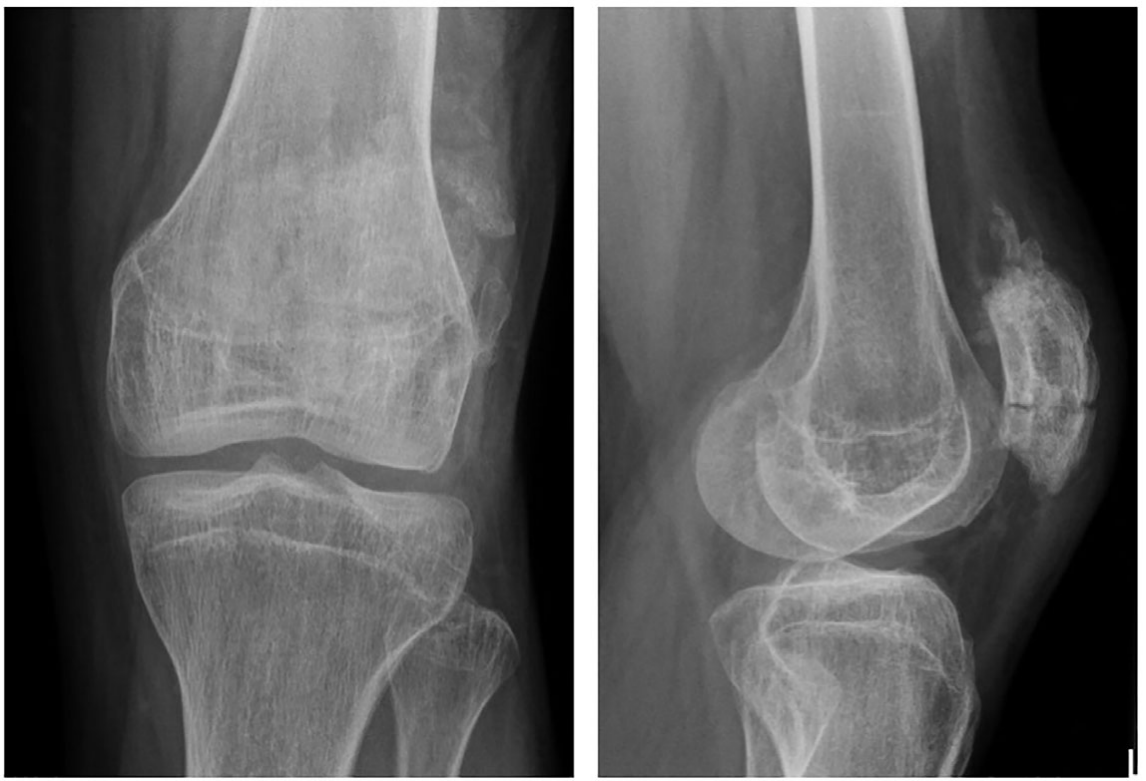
AP and lateral radiographs showing a transverse fracture of the left patella.
